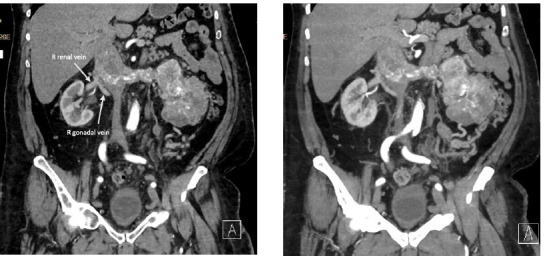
Preoperative right renal venous drainage. Preoperative coronary computed tomographic (CT) angiography of the abdomen and pelvis showing the right gonadal vein in close proximity to the right renal vein as well as tumor originating from the left kidney and suprarenal invasion of the inferior vena cava.
